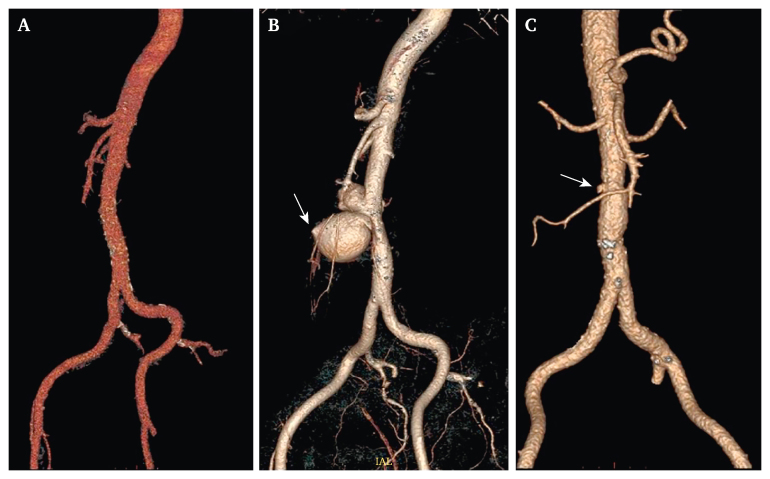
Three dimensional reconstructions of the evolution of the double aorto-enteric fistula (AEF). (A) A reconstruction of the aorta six months prior to the primary aorto-enteric fistula. (B) Imaging taken at the clinical presentation of the primary AEF; the scan shows a clear saccular aneurysm with extravasation to the duodenum (arrow). (C) Pseudoaneurysm (arrow) on the latest computed tomography scan available two weeks pre-operatively for the secondary AEF (focused on the latter secondary AEF).
